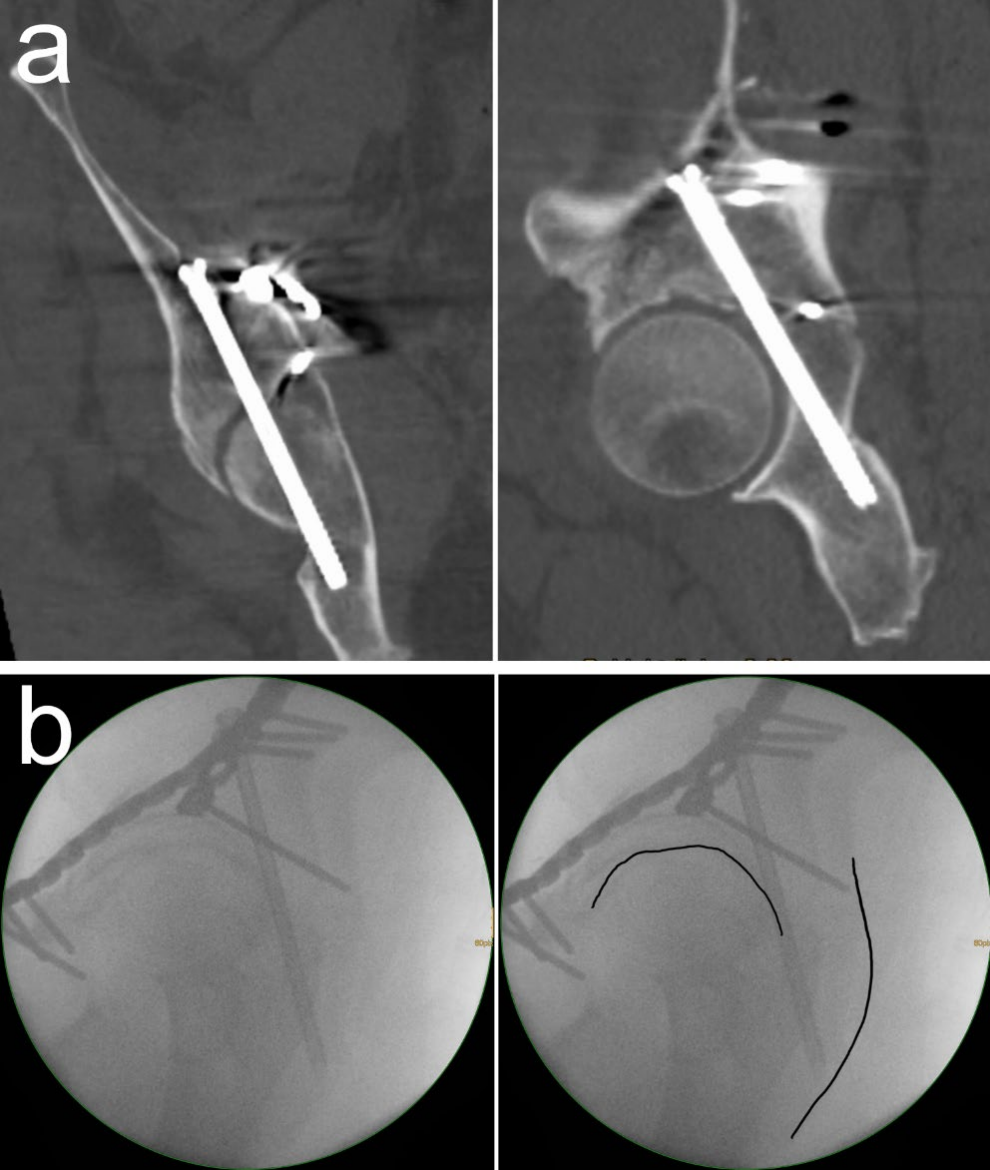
Hip joint penetration. a Postoperative CT scan of an ACPHT fracture treated with PCS showing adequate reduction of the posterior hemitransverse fracture and no posterior cortical perforation of the posterior column, but hip joint penetration. Coronal reconstruction (left) and sagittal reconstruction (right). b The lateral oblique view shows the close proximity of the PCS to the hip joint despite poor image quality and poor side differentiation
